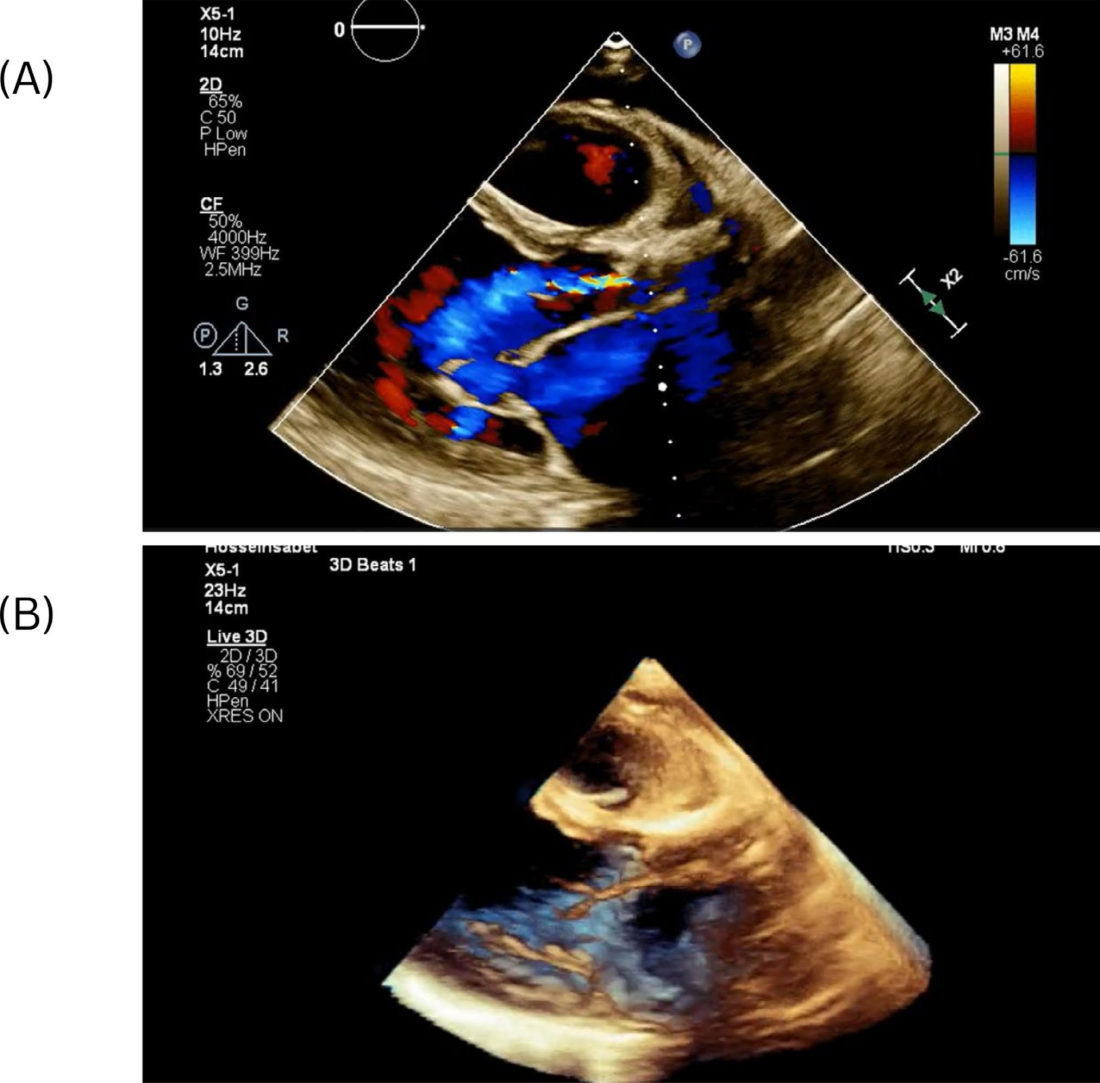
(A) 2D color Doppler echocardiography of the parasternal view showing supravalvular AS. (B) 3D echocardiography of the parasternal view demonstrating the same supravalvular AS.
